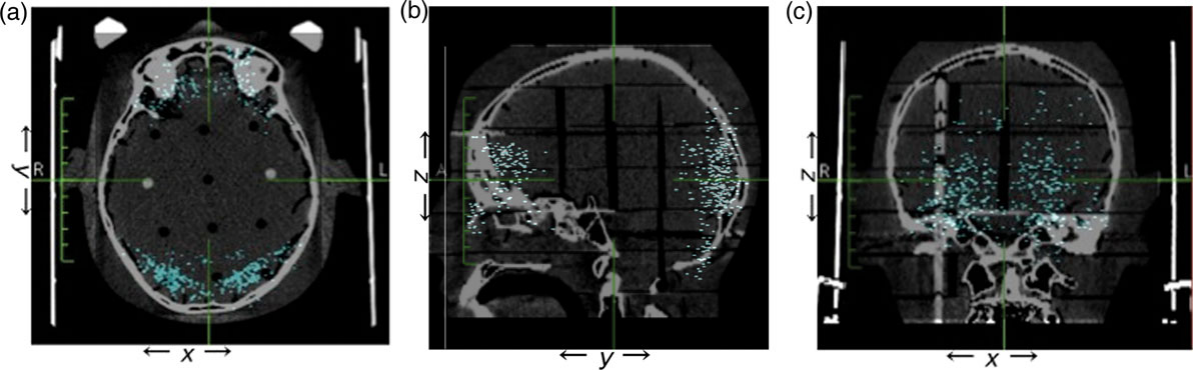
Axial (a), sagittal (b), and coronal (c) computed tomography images on which clearance-warning coordinates obtained from 177 patients, 213 lesions and 461 isocenters treated by PFX were superimposed.
